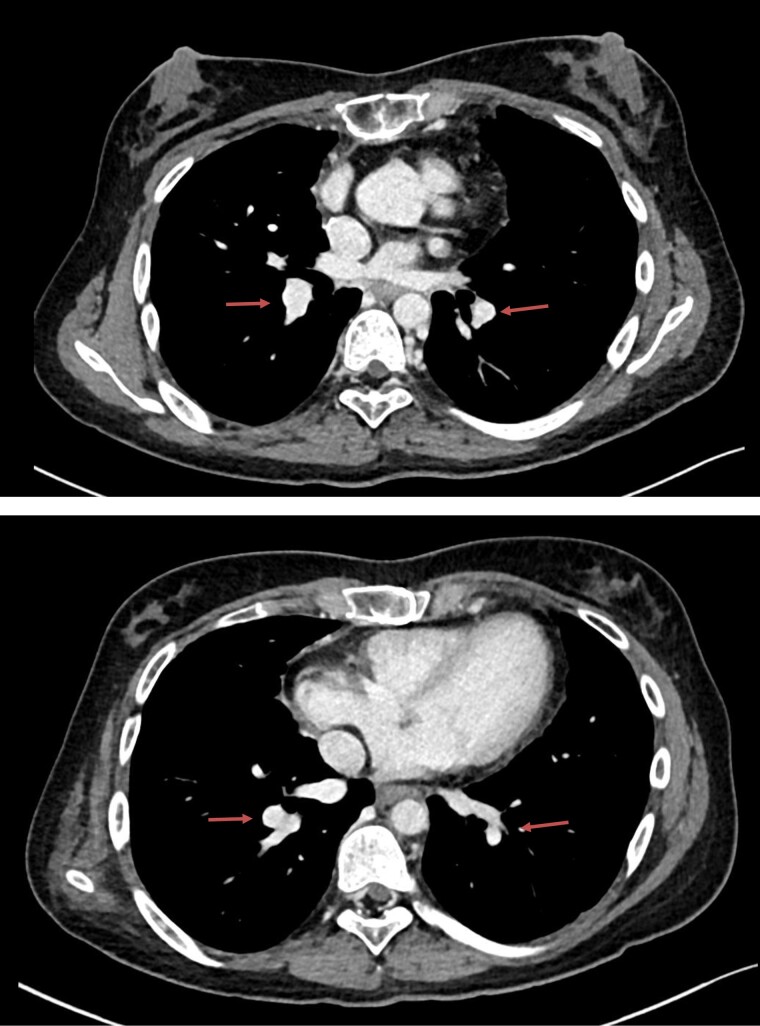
Post percutaneous thromboaspiration control chest computed tomography with resolution of pulmonary thromboembolism (arrows).
